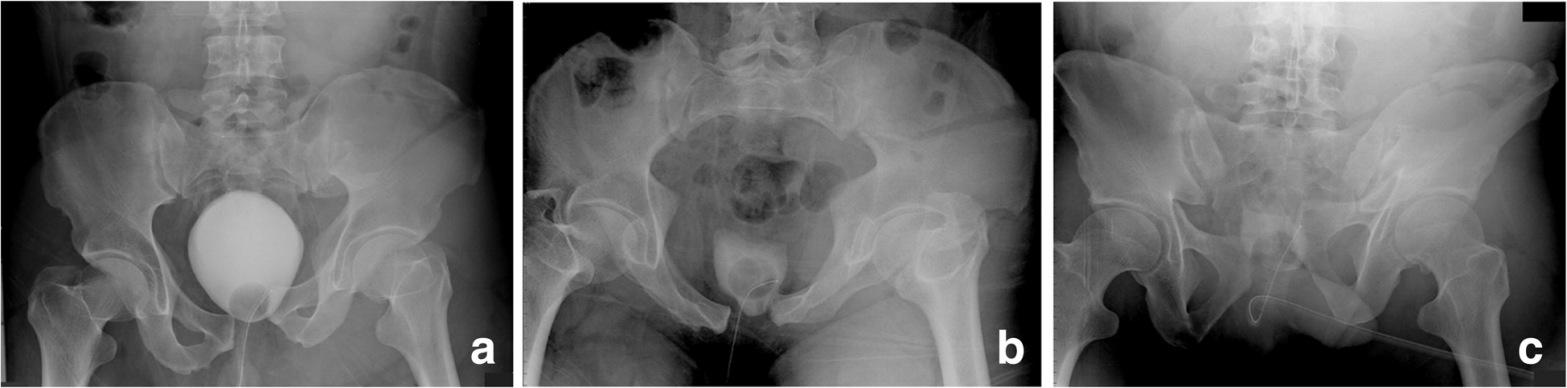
Conventional plain AP (a), inlet (b) and outlet (c) pelvic radiographs obtained on the day of injury in a polytrauma patient
